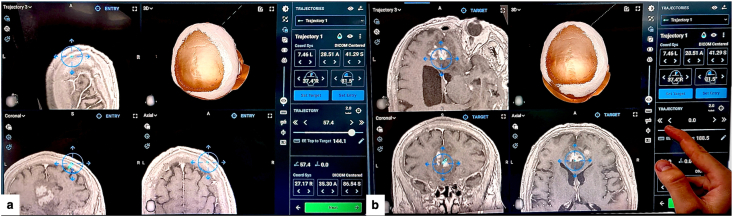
Robotic planning of the stereotactic trajectory (ExcelsiusGPS). (a) CT-MRI fusion and 3D reconstruction showing a right frontal entry point providing the shortest safe, near-perpendicular corridor avoiding eloquent cortex and superficial vessels. (b) Target positioned within the most representative component of the lesion, defining a coaxial trajectory through a micro-burr hole of approximately 57.4 mm.
